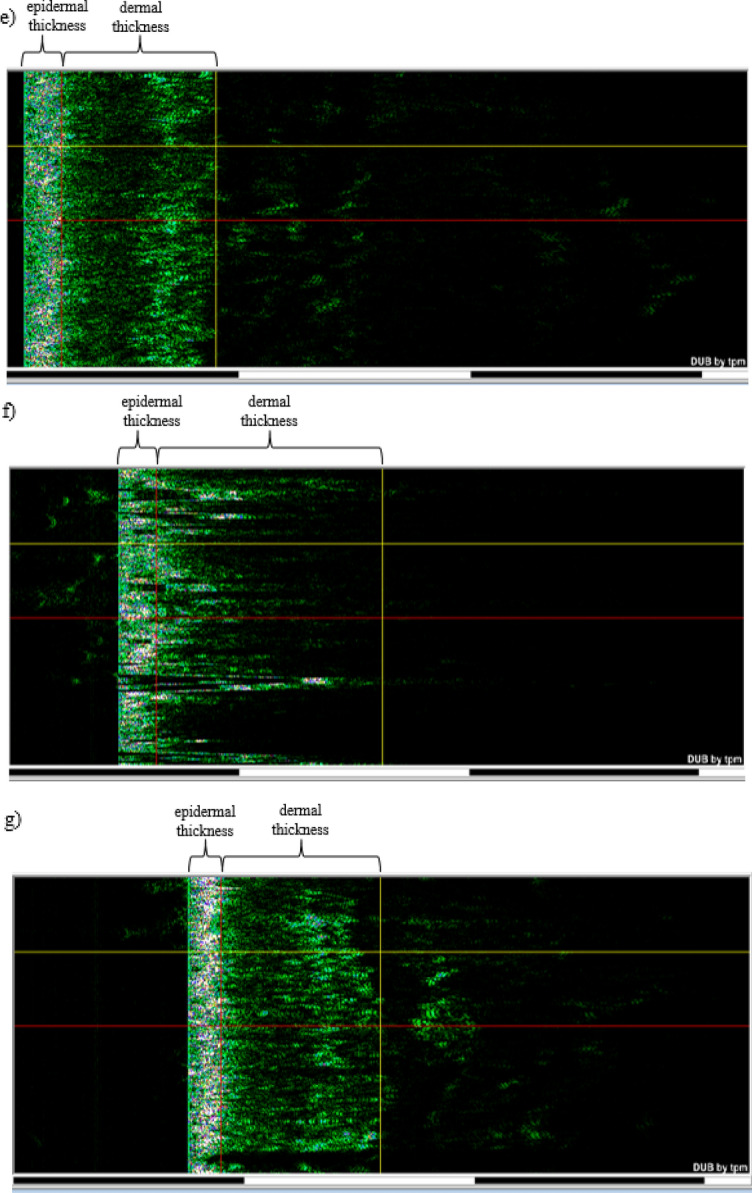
Skin thickness and density were obtained using ultrasound. Dermis is displayed from a red to a yellow vertical line, and the epidermis is indicated from a green to a red vertical line. Plasma group: (a) before, (b) immediately after treatment, (c) 2 weeks later, (d) 4 weeks later. Control group: (e) before the experiment, (f) after 2 weeks, (g) after 4 weeks.
